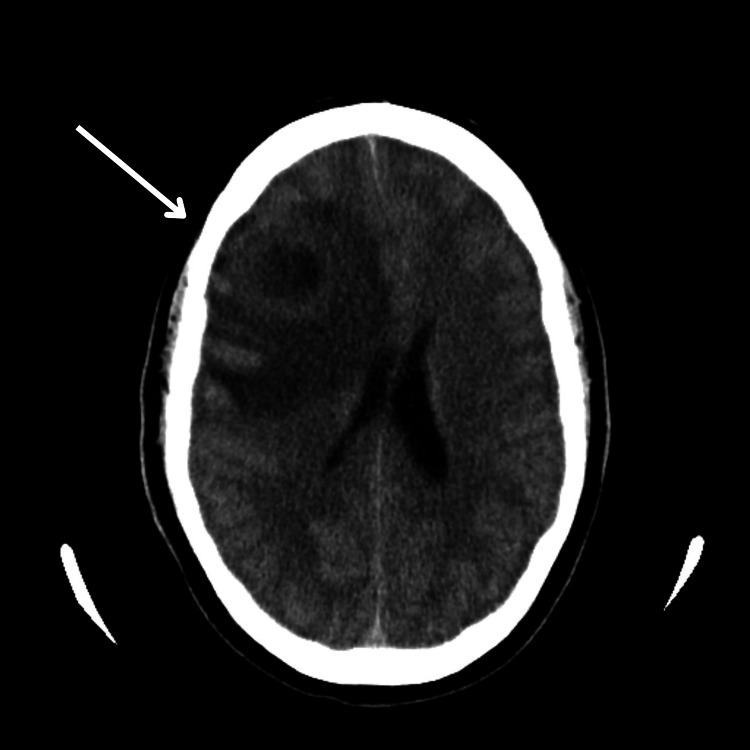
Preoperative head imaging CT head showing a 3.8 x 1.6 cm right frontal lobe abscess with a 7 mm leftward midline shift.
